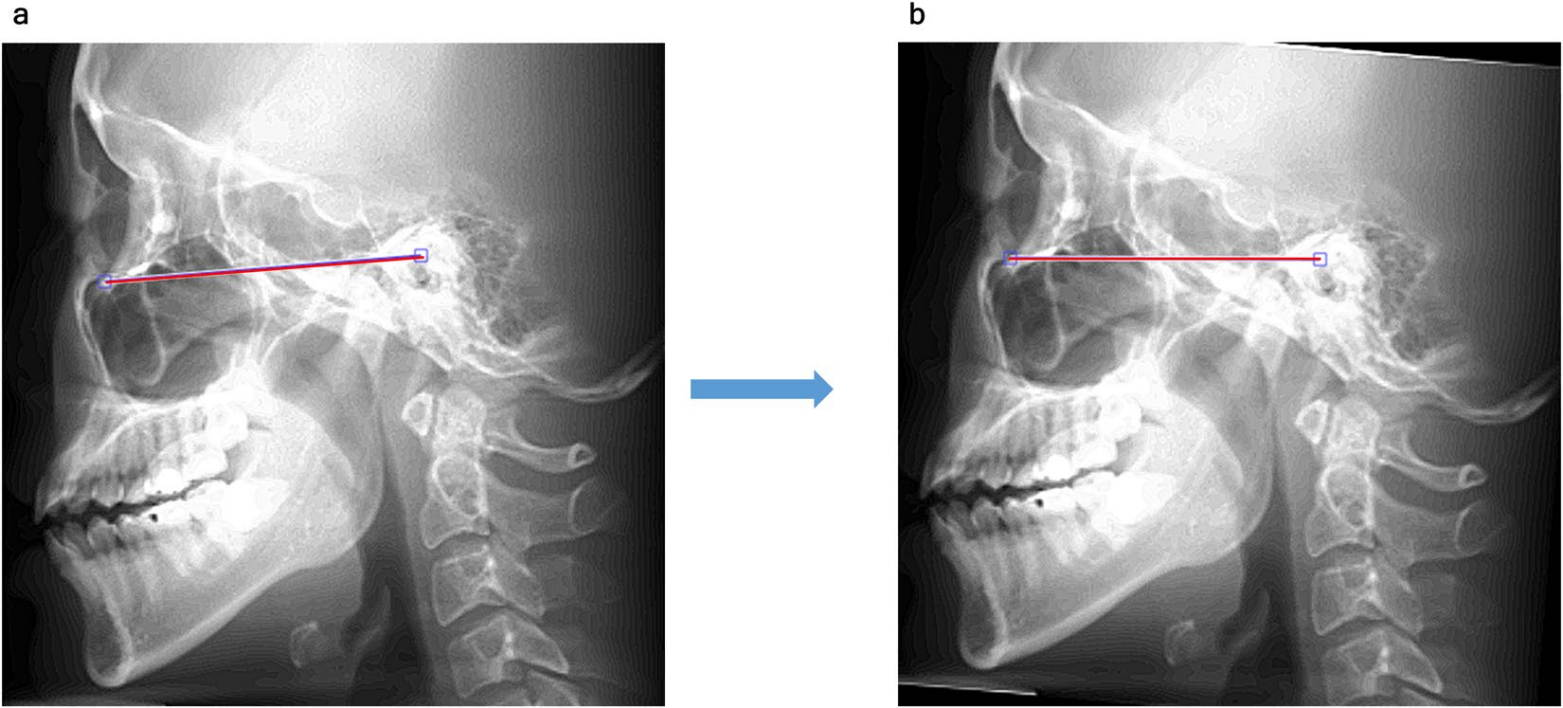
Image reorientation (sagittal). (a) Using the average intensity projection image in the sagittal direction, a Frankfort plane (red segment) was drawn passing through the 2 points defined by the inferior margin of the orbit and the upper margin of the external auditory meatus. (b) The entire image was rotated around the center of the two points for the Frankfort plane to be parallel with the floor.The entire 3D image was rotated around the center of the 2 points for the Frankfort plane to parallel with the floor (Fig. 2b). This procedure aligns the position of the head in the image for panoramic radiography.
